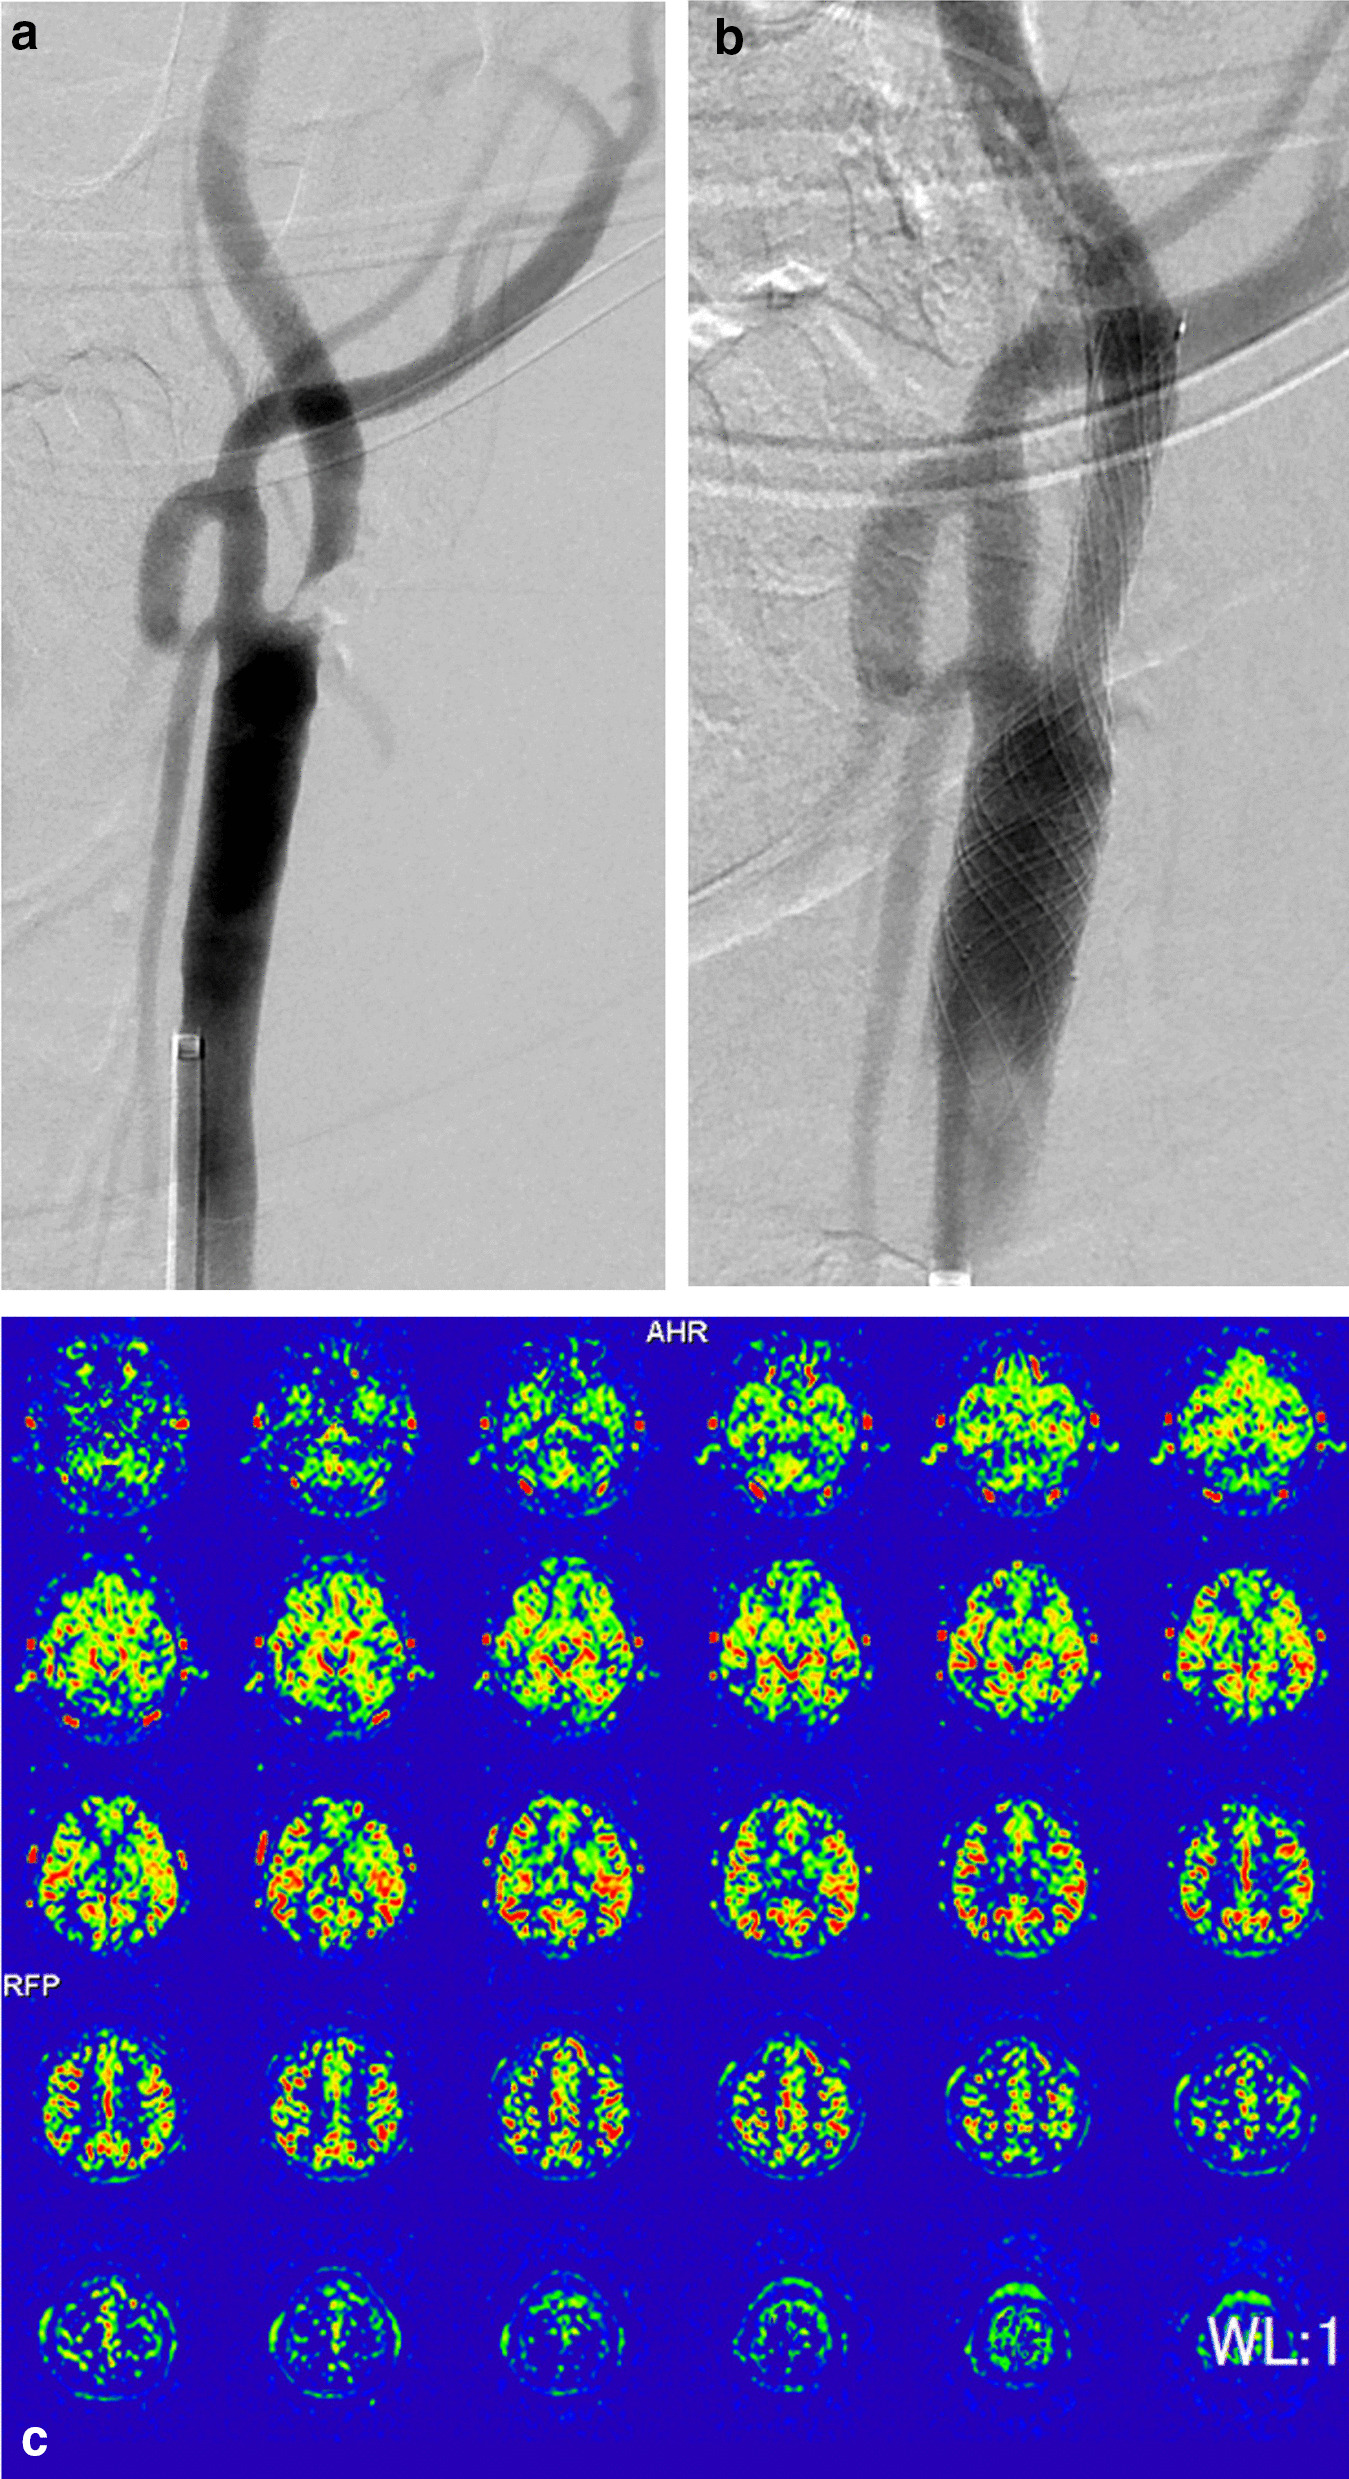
a Preprocedural carotid angiography showing severe stenosis of the left common carotid artery. b Postprocedural carotid angiography showing improvement of the severe stenosis of the left common carotid artery. c Postprocedural arterial spin labeling of the magnetic resonance imaging showing improvement of the low perfusion areas in the left lobe
